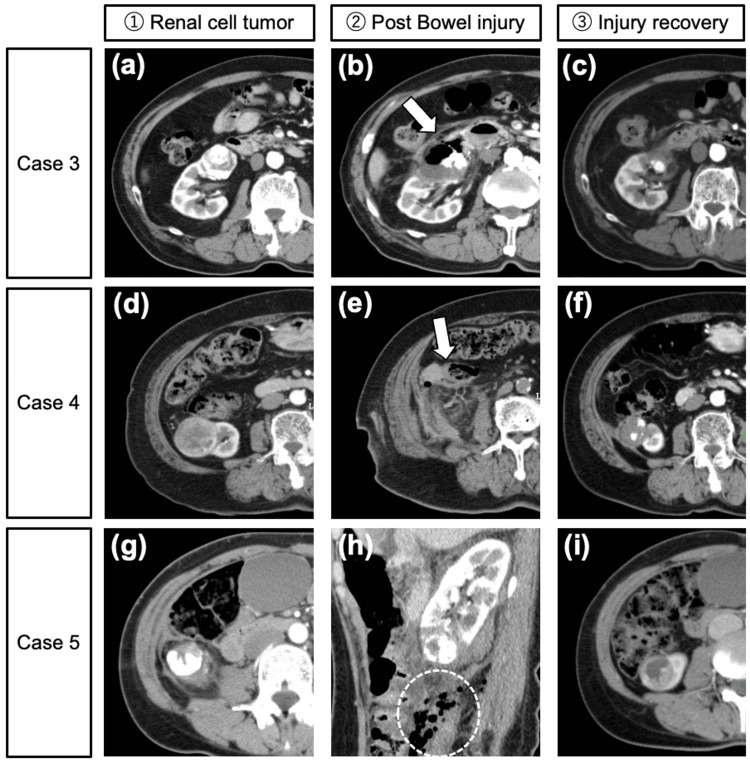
Cases 3-5: Bowel injuries following PCA Case 1: Duodenal Fistula (a) Preoperative image showing a 39 mm ccRCC in the right kidney. (b) Post-PCA CT image demonstrating a duodenal fistula (arrow). (c) The fistula spontaneously closed without the need for intervention. Case 2: Ascending colon injury (d) Preoperative image showing a 53 mm mass in the right kidney. (e) CT image demonstrating thickening of the colon wall (arrow) suspected to be due to needle injury during hydrodissection. (f) The complication spontaneously resolved with bowel rest. Case 3: Descending colon injury (g) Preoperative image showing a 30 mm mass in the right kidney. (h) Post-PCA CT image showing perinephric and colonic air (dotted circle). (i) Spontaneous resolution with conservative management. PCA: percutaneous cryoablation
